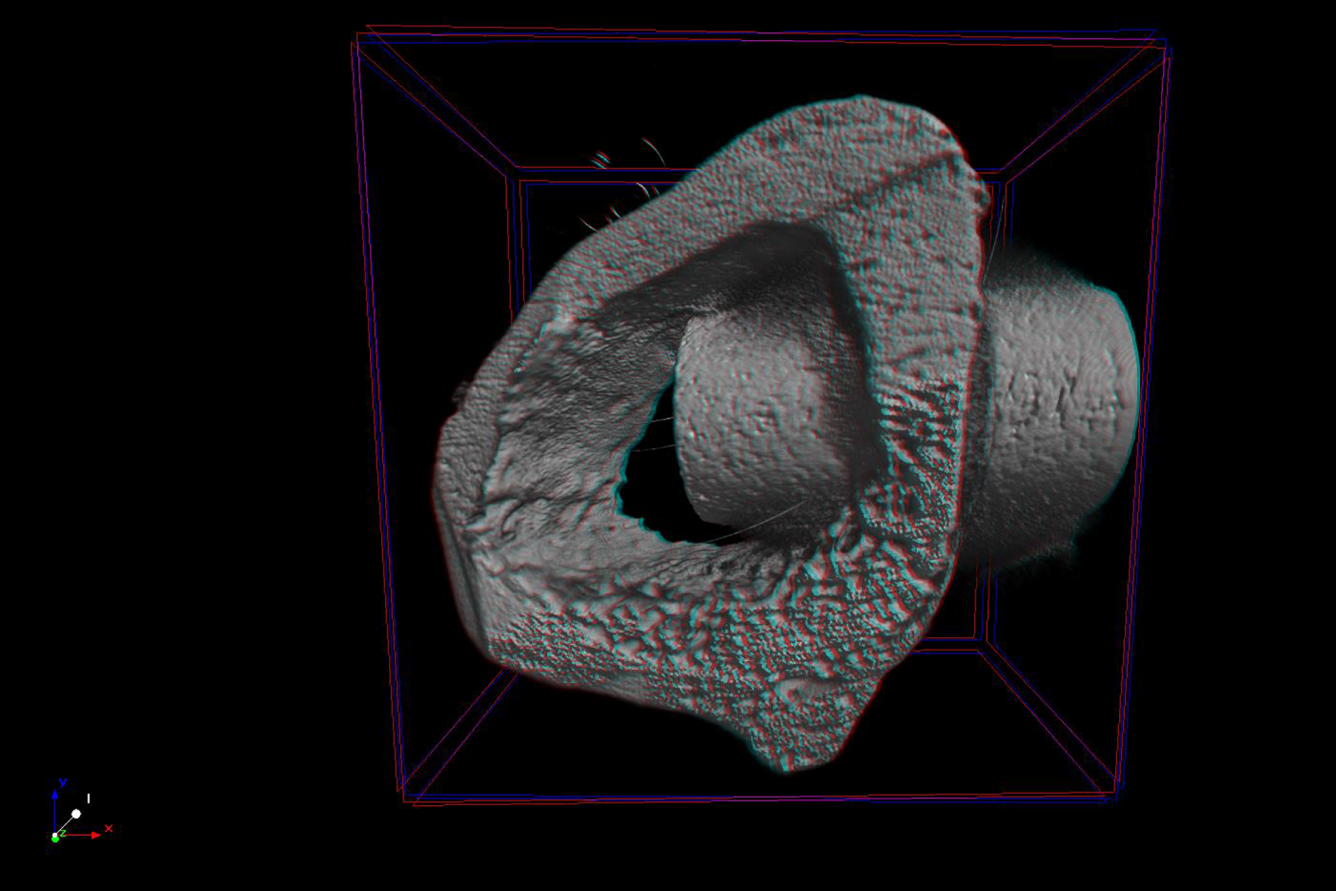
3D representation of micro-computed tomography. Image of implant and bone around implant at 4 weeks.
